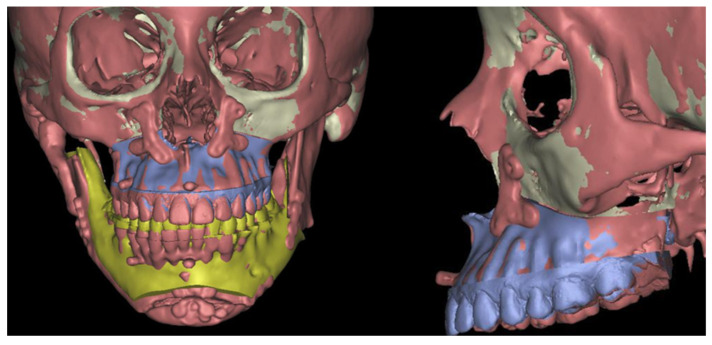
Superimposition analysis of preoperative virtual planning and post-operative CT scan.
